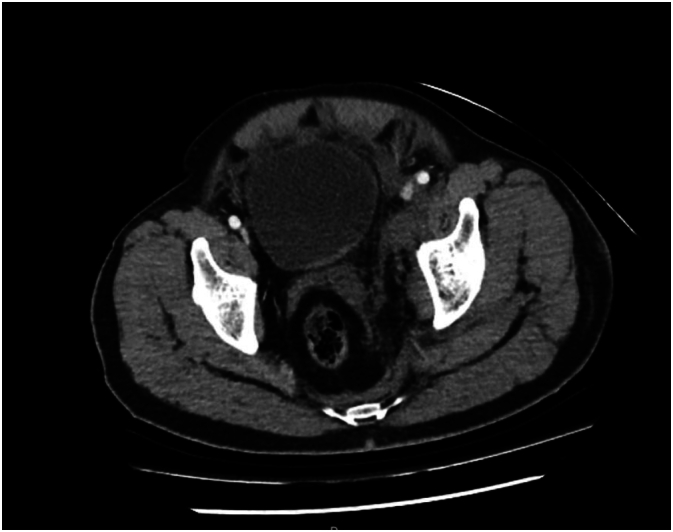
Axial CT Pelvis with inflated bladder and non-enhancing left hematoma.
